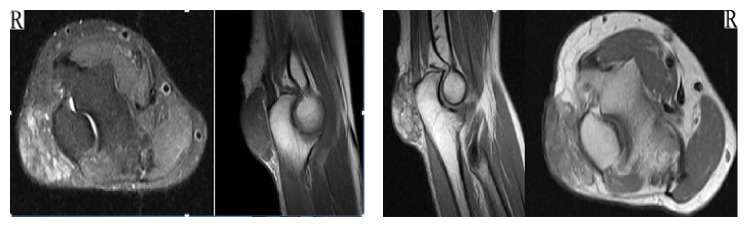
Preoperation right elbow MRI images, T1a and T2a sections, at site of the posterior bursa of 44 × 21 × 32 mm sized complicated tissue. No destruction of bone (9/5/16).
